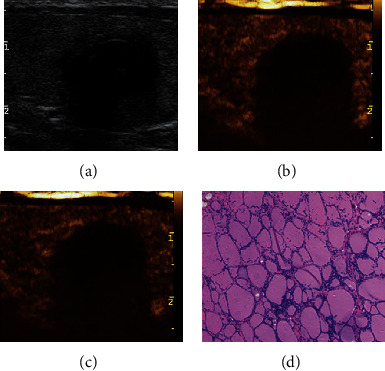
Adjustment of C-TIRADS classification of thyroid nodule with no enhancement in CEUS. A 63-year-old male patient with a right thyroid nodule, maximum diameter of 20 mm, which was originally classified as C-TIRADS 4C before the adjustment based on the CEUS results. The nodule was reclassified as C-TIRADS 3 after the adjustment. (a) Two-dimensional gray scale. (b) No enhancement at 17 s in CEUS. (c) No enhancement at 37 s in CEUS. (d) Diagnosed as nodular goiter combined with collagen degeneration and focal necrosis (HE × 100). CEUS: contrast-enhanced ultrasound; C-TIRADS: the Chinese-Thyroid Imaging Report and Data System.
